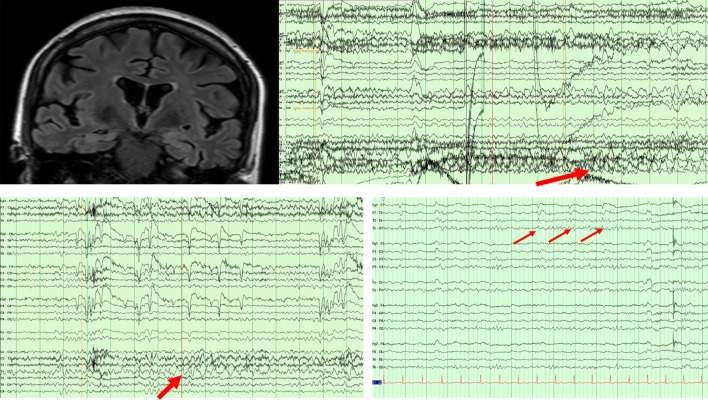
MRI FLAIR sequence revealing bitemporal FLAIR hyperintensities in patient 1. EEG (above) showing a right temporal seizure in patient 1. EEG (below left) showing left temporal rhythmic delta activity in patient 1. EEG (below right) showing periodic left temporal sharp waves in patient 2.
